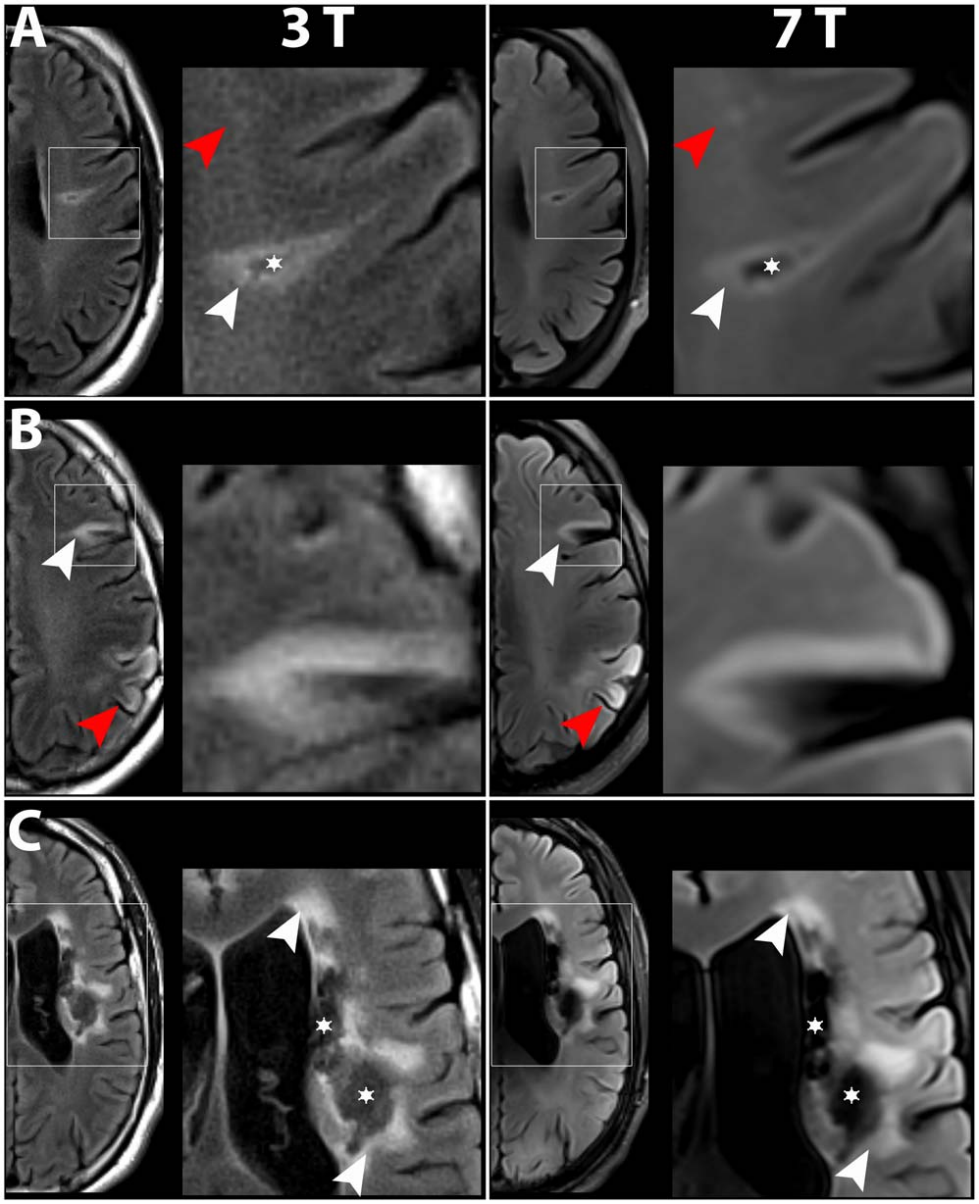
Comparison of T2-FLAIR images obtained at 3 T and 7 T. In all patients, all lesions detected at 3 T were also visible at 7 T. Boxed areas are shown at higher magnification. A) Patient No. 3, with a small chronic lesion consisting of hyperintense postischemic tissue (white arrowheads) surrounding a tissue defect area (asterisk); compare also figure 2 A. At 7 T, the intensity values of the tissue defect area were comparable to CSF, while at 3 T, the intensity values were comparable to white matter. Contrast between postischemic and healthy brain tissue was higher at 3 T. However, small white matter lesions (red arrowheads) were easier to identify at 7 T. B) Patient No. 1, with a chronic stroke lesion (white arrowheads) and a subacute lesion (red arrowheads). Both lesion types were readily identifiable at both field strengths. As in A), contrast between the lesion and healthy tissue appeared to be higher at 3 T. C) Patient No. 4, with a large chronic infarct, consisting of hyperintense lesion areas (white arrowheads) and hypointense defect areas (asterisks). Again, CSF-filled tissue defect areas were easier to identify at 7 T, while the lesion to healthy tissue contrast was higher at 3 T. Compare also fig. 2 A–C.
